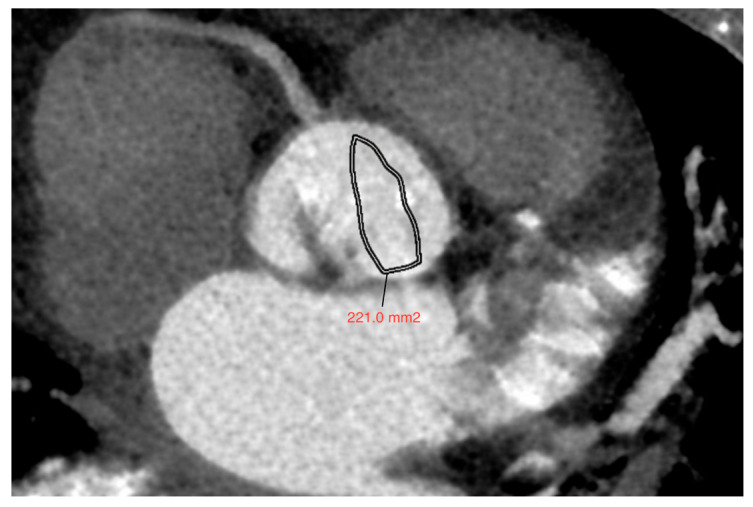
A 67-year-old female patient with a bicuspid aortic valve. The aortic valve area (AVA_CT_) calculated in computed tomography—221 mm^2^ was similar to the area obtained using the proposed regression model.
